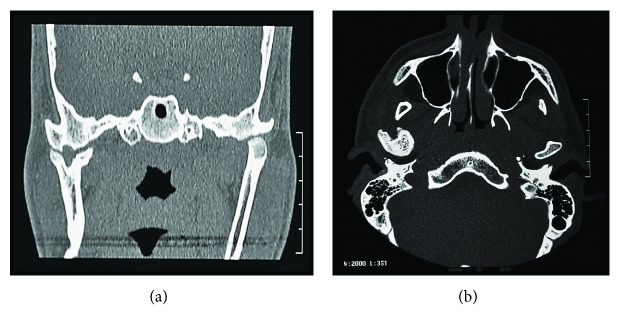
(a) Coronal view of the CT scan emphasizing the bifid mandibular condyle in the laterolateral direction. (b) Axial view of the CT scan showing ankylosing mass.
